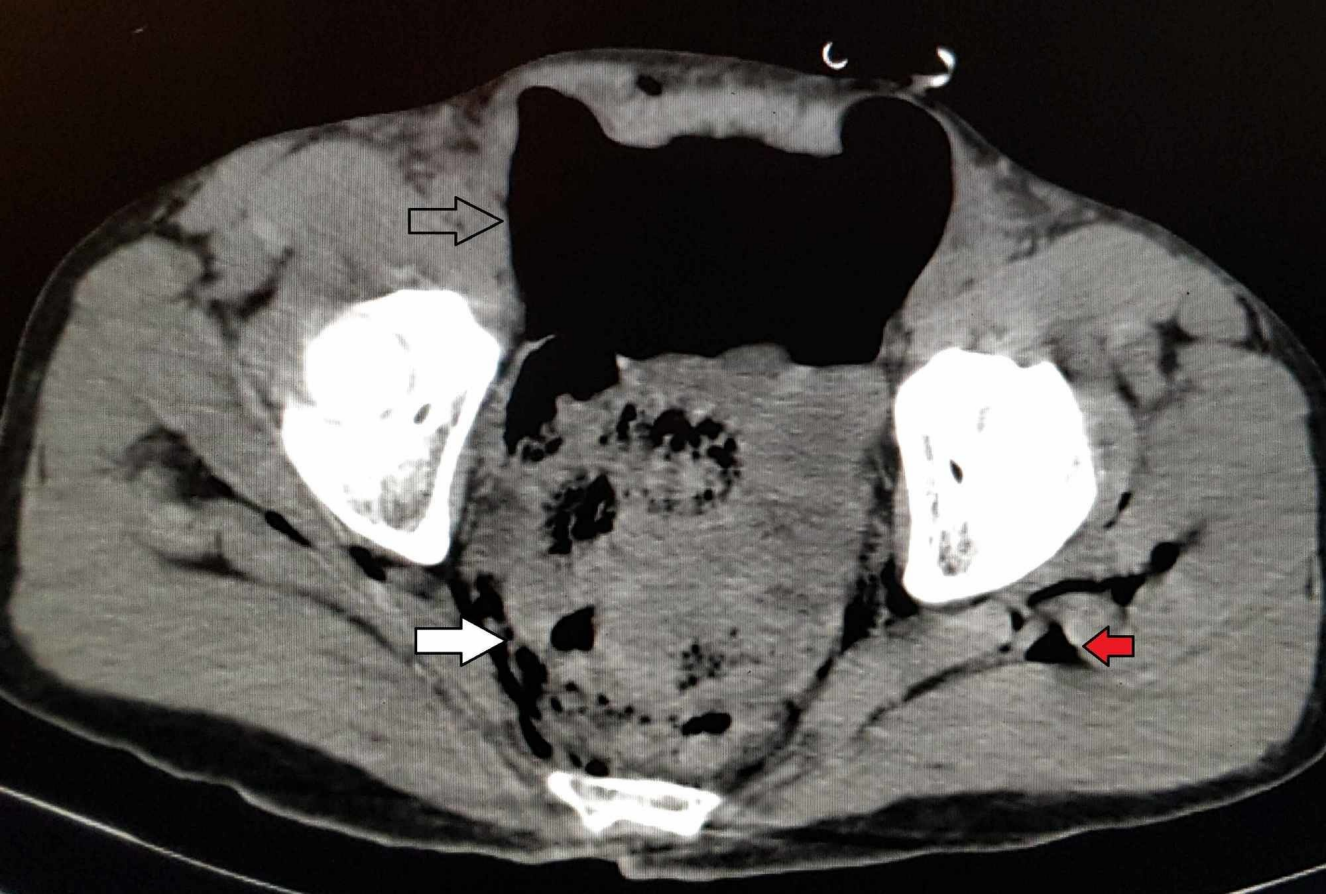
CT of the pelvis showing the pneumoperitoneum (arrow), air in the mesorectum (white arrow) and subcutaneous emphysema (red arrow).
